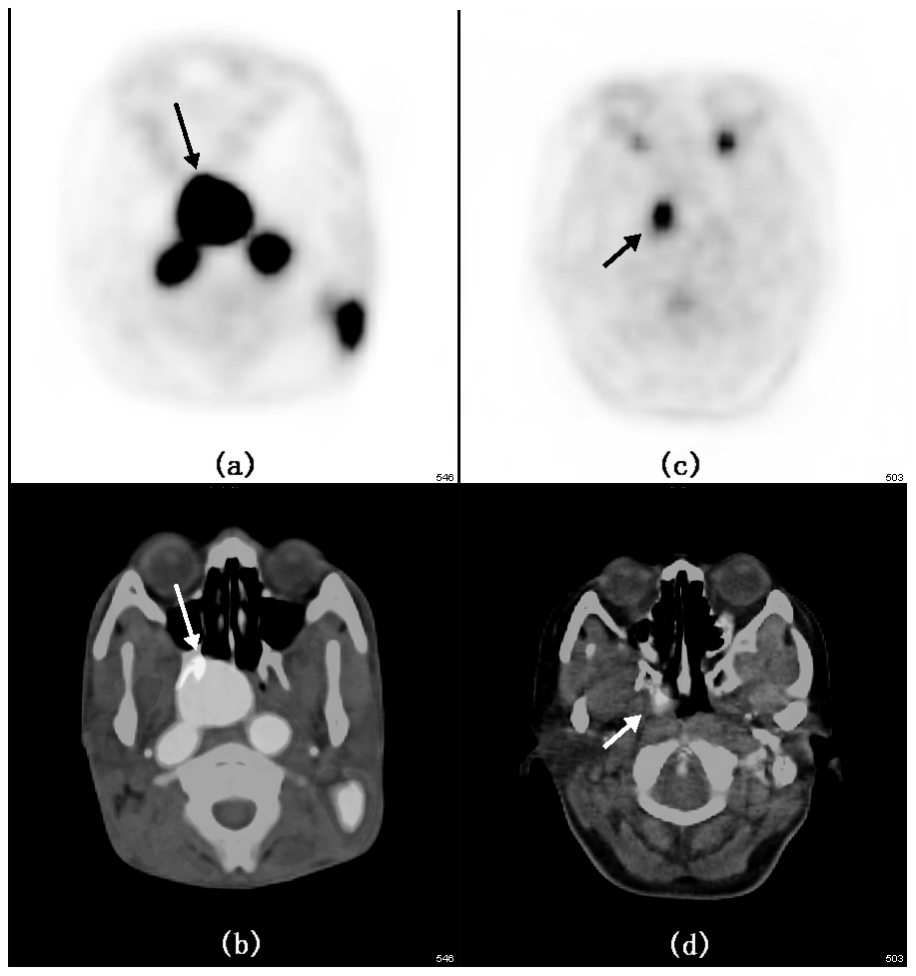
(a) and (b) were PET image and PET/CT infusion image respectively, showing the primary lesion of a patient with T3N1M0 disease, with SUVmax-T (maximal standardized uptake value of primary tumor) of 20.30 and Total SUVmax (sum of maximal standardized uptake value of primary tumor and cervical lymph nodes) of 58.73. This patient developed liver metastasis 8 months after treatment. (c) and (d) were PET image and PET/CT infusion image respectively, showing the primary lesion of a patient with T1N0M0 disease, with both SUVmax-T and Total SUVmax of 6.71. Neither recurrence nor distant metastasis has happened in this patient till now. The arrows pointed after the primary lesions with increasing uptake of FDG.
